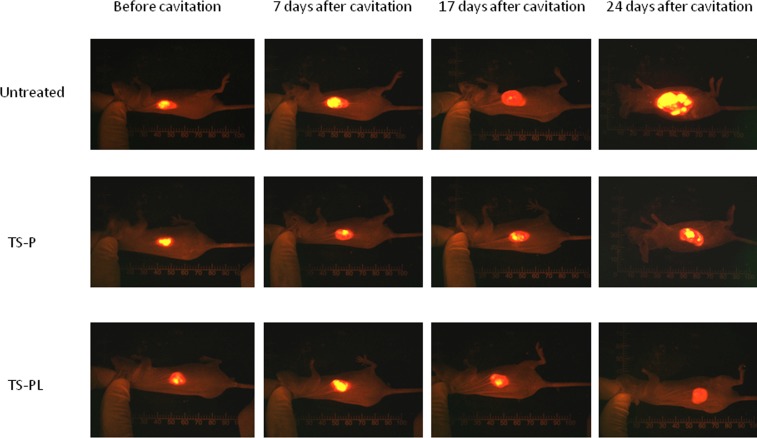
Inhibitory effect of Up-sized MB on xenograft tumor growth Whole body fluorescence imaging was performed to measure tumor size at different time points during the study as described in the MATERIALS AND METHODS. Sequential in vivo real-time fluorescence imaging of tumor progression after ultrasonic cavitation.
